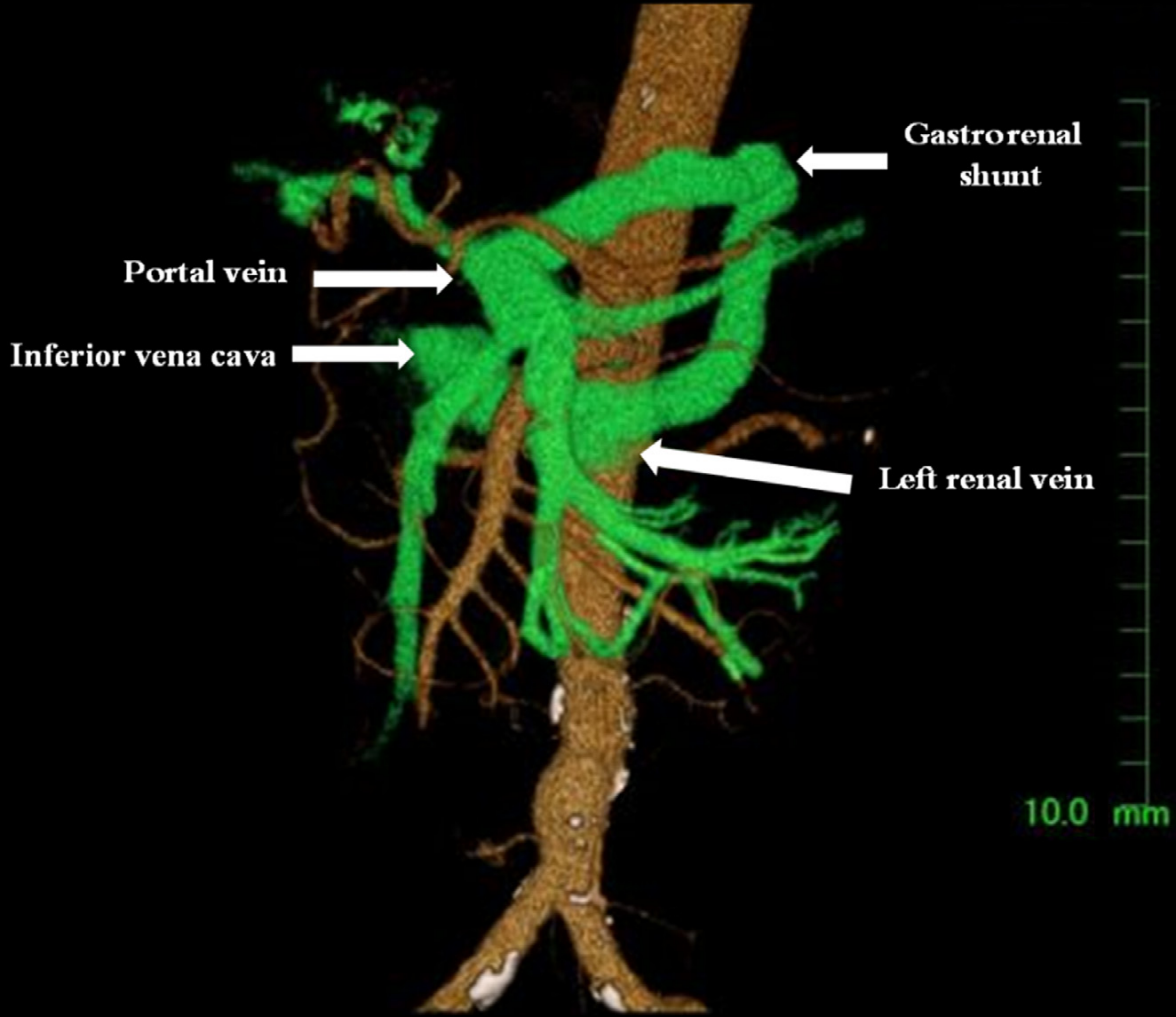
Gastrorenal shunt: Three‐dimensional reconstruction of abdominal‐enhanced computed tomography image.
